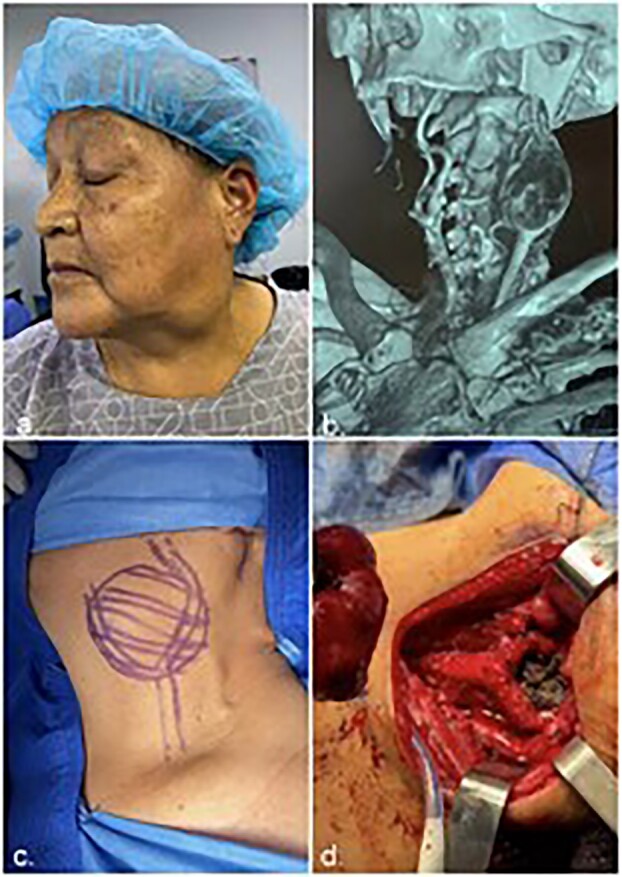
(a) Patient with palpable neck mass (carotid body tumor), (b) CT scan of a patient from the case series, (c)draft of Shamblin classification and (d) observation of CBT commitment.
